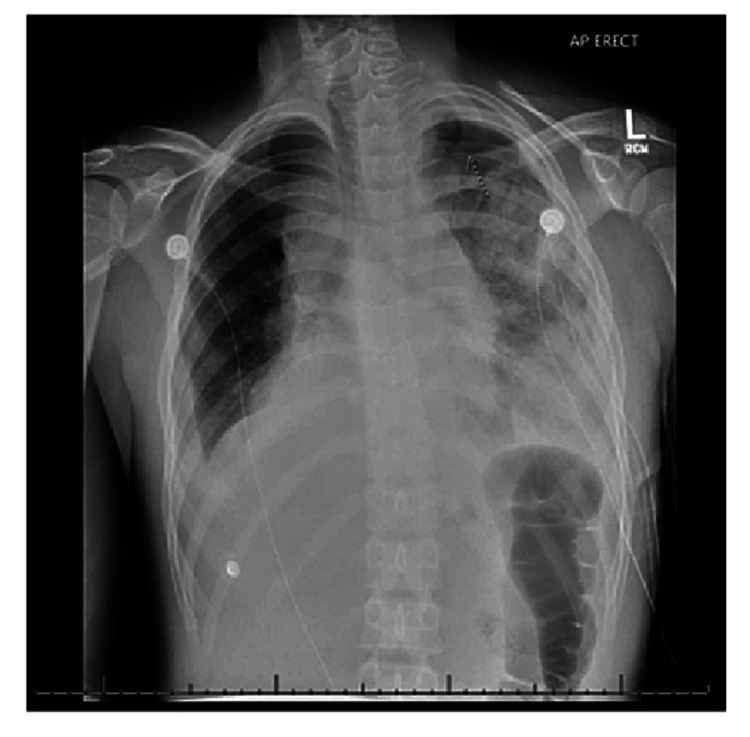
Chest x-ray (AP) view was done after the chest tube was placed and fluid drained showing enlargement of the left hilum pulmonary hilum and AP window region, raising the possibility of mediastinal mass. AP = anterior-posterior
